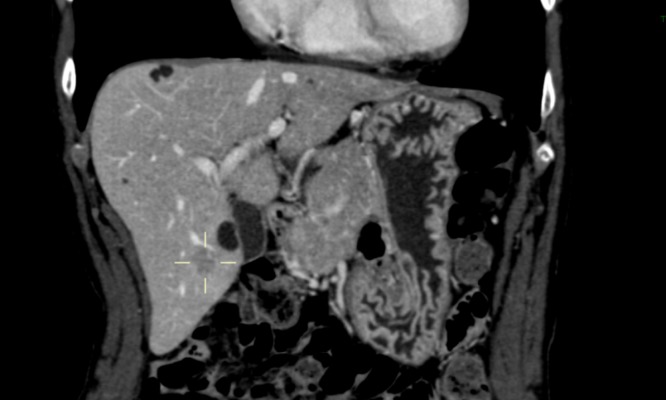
First diagnostic CECT imaging (April 2017) Cystic lesions (with arrows) and V segment metastasis (grey arrow). contrast-enhanced computed tomography: CECT
